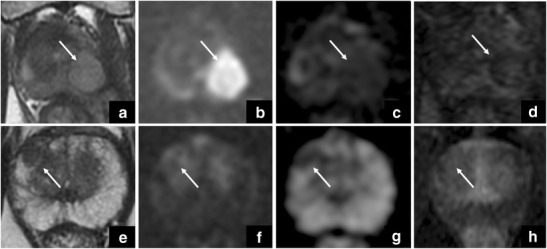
The first four images show a round-shaped area characterised by intermediate signal intensity on T2-weighted imaging (a), restricted diffusion on DWI (b) and in the ADC map (c), and ring enhancement (d). These findings, together with clinical history, orient towards the diagnosis of abscess. The other four images show a focal nodule in the right anterior peripheral zone, characterised by low signal intensity on axial T2-weighted imaging (e) with sharply defined margins and tiny bright spots, and restricted diffusion on DWI (f) and in the ADC map (g), and homogeneous enhancement on DCE imaging (h). These findings are consistent with an ectopic nodule of BPH
